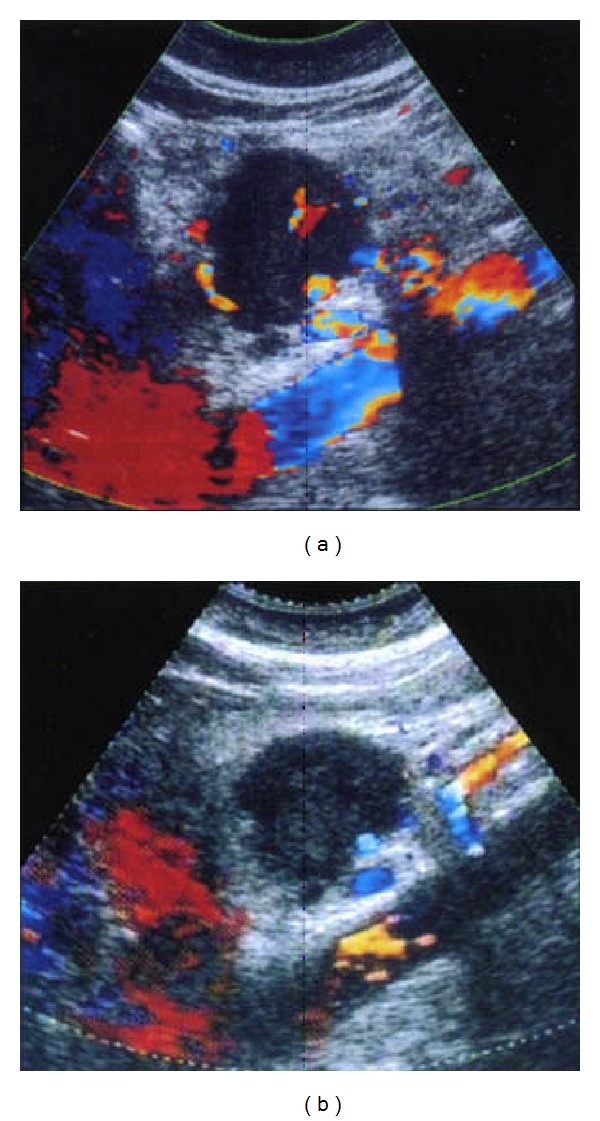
Sonography of pancreatic cancer (a) before and (b) after HIFU therapy showing the enhancement of echogenicity in the tumor but decrease of vascularity, an indicator of coagulative necrosis (used with permission [20]).
